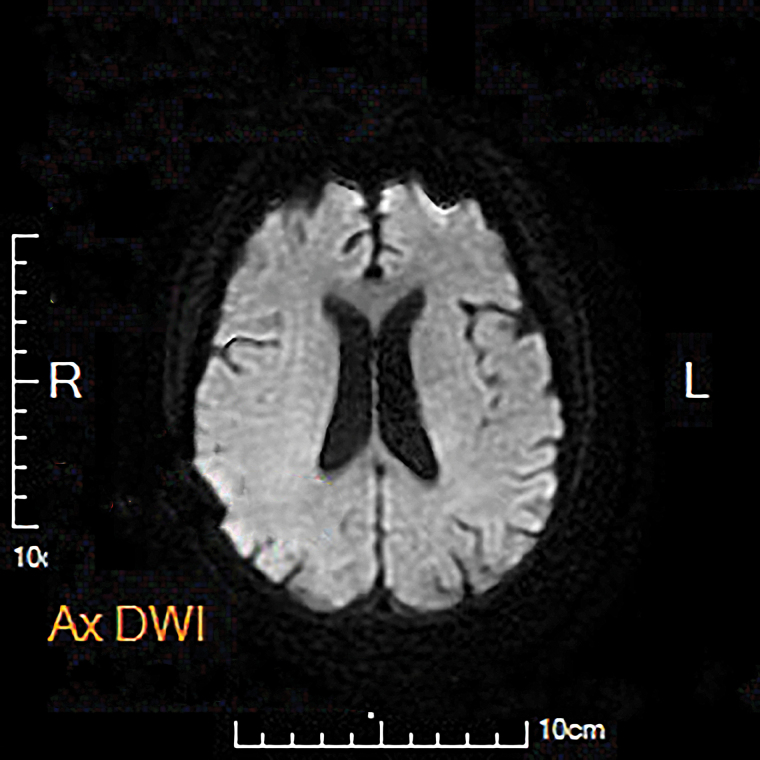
MRI diffusion-weighted image shows no acute changes. MRI = magnetic resonance imaging.
